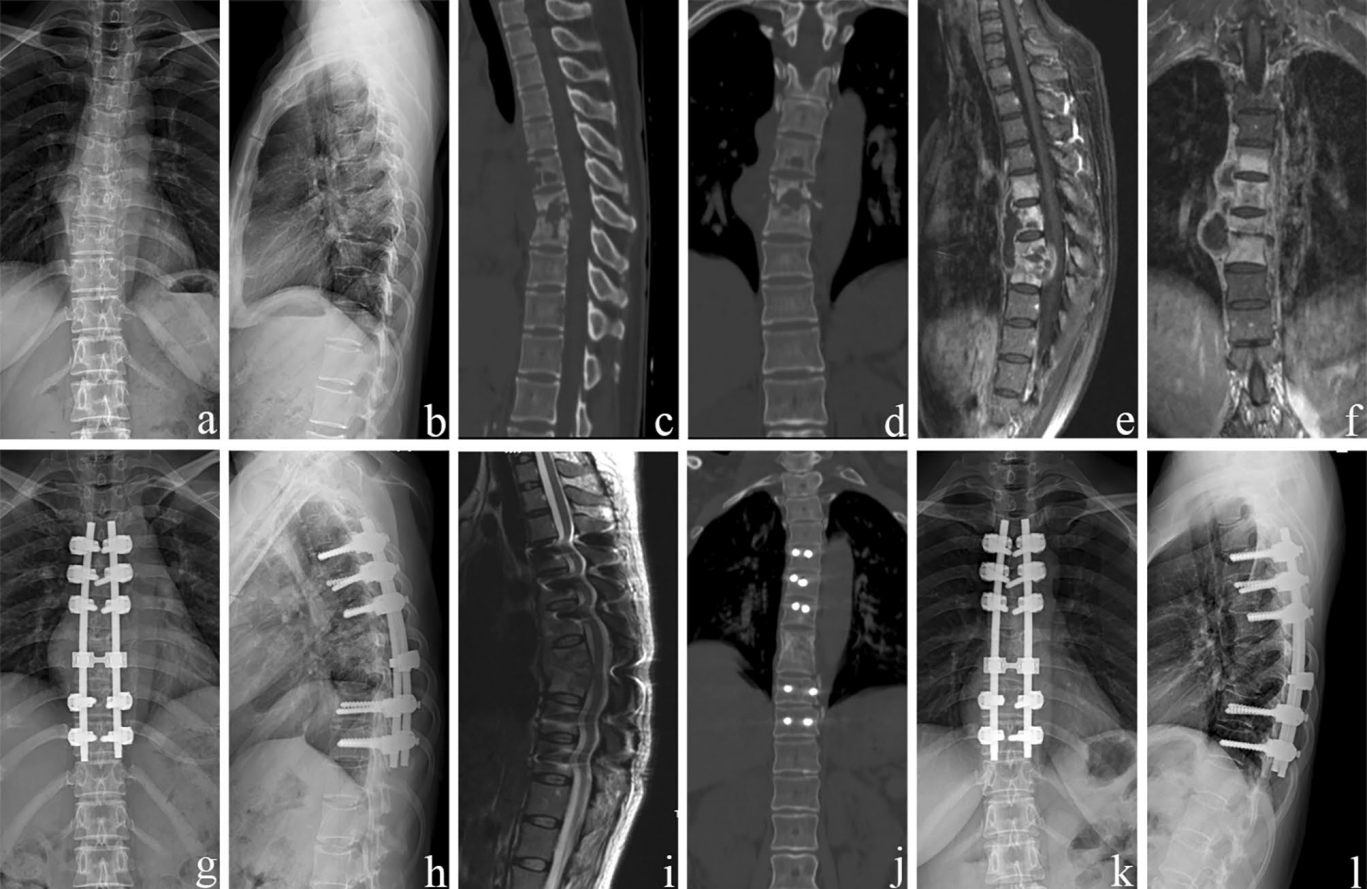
A 49-year-old female demonstrating lesions received a one-stage posterior transforaminal thoracic debridement, interbody fusion, and instrumentation. (a–f) Preoperative radiographs, CT, and MRI scans show TB of T5–T8 with vertebral bone destruction and deformity (kyphosis angle 36°) and paravertebral abscess formation. (g,h) Postoperative radiographs demonstrate the correction of the deformity (kyphosis angle 23°). (i) Postoperative MRI that shows clearance of TB lesions and spinal cord decompression. (j) CT shows satisfactory bone fusion at 12 months. (k,l) Radiographs display good internal fixation position and solid bone fusion, with a correction loss of 1° after 81 months of follow-up.
